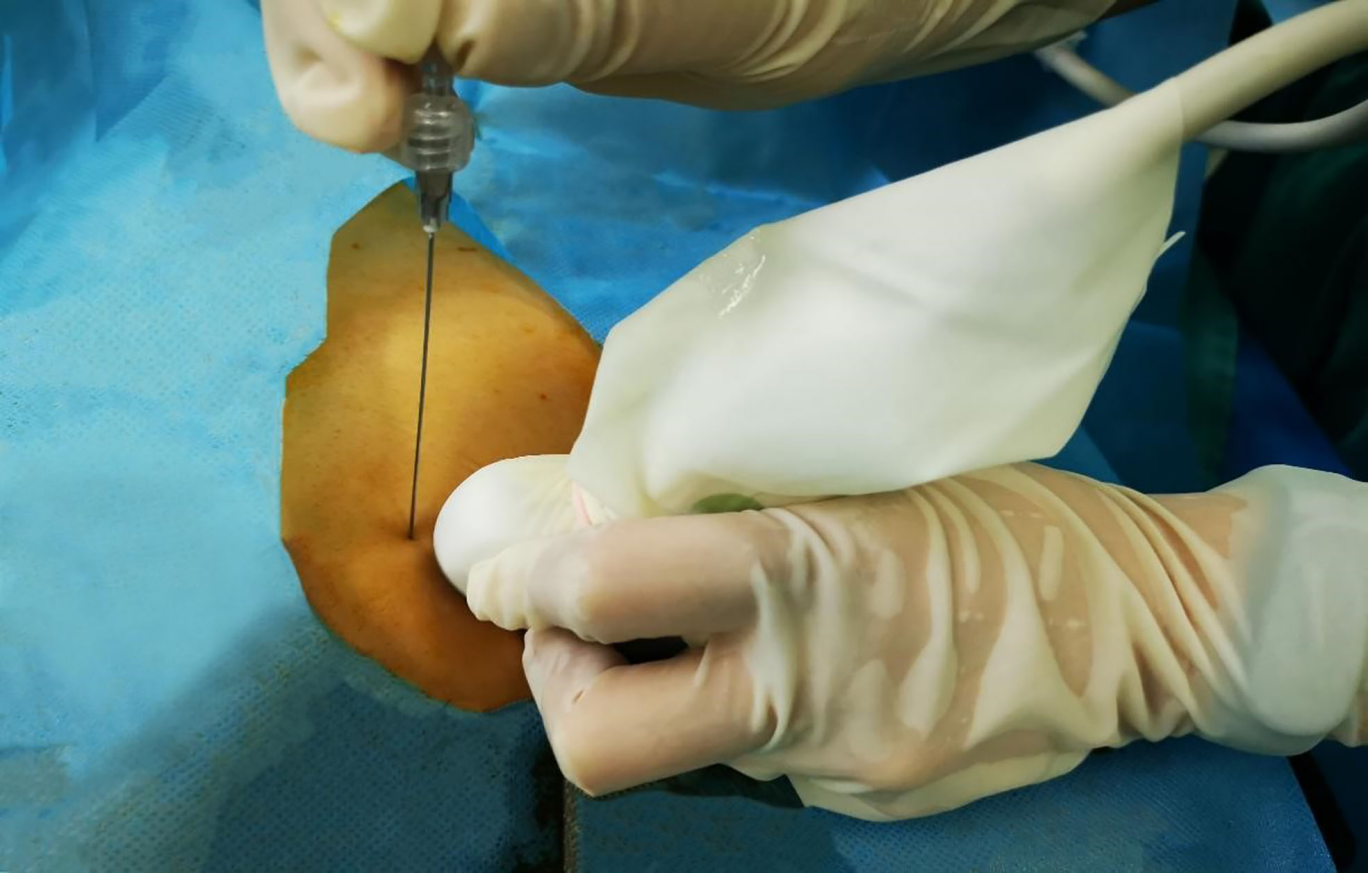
Ultrasound-guided paravertebral puncturing.
